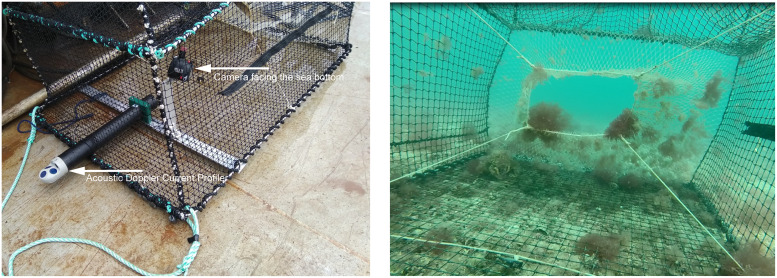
Position of the camera facing the sea bottom and of the Acoustic Doppler Current Profiler (left panel) and View of the bottom from the camera set to quantify the swept distance during retrieval (right panel).
